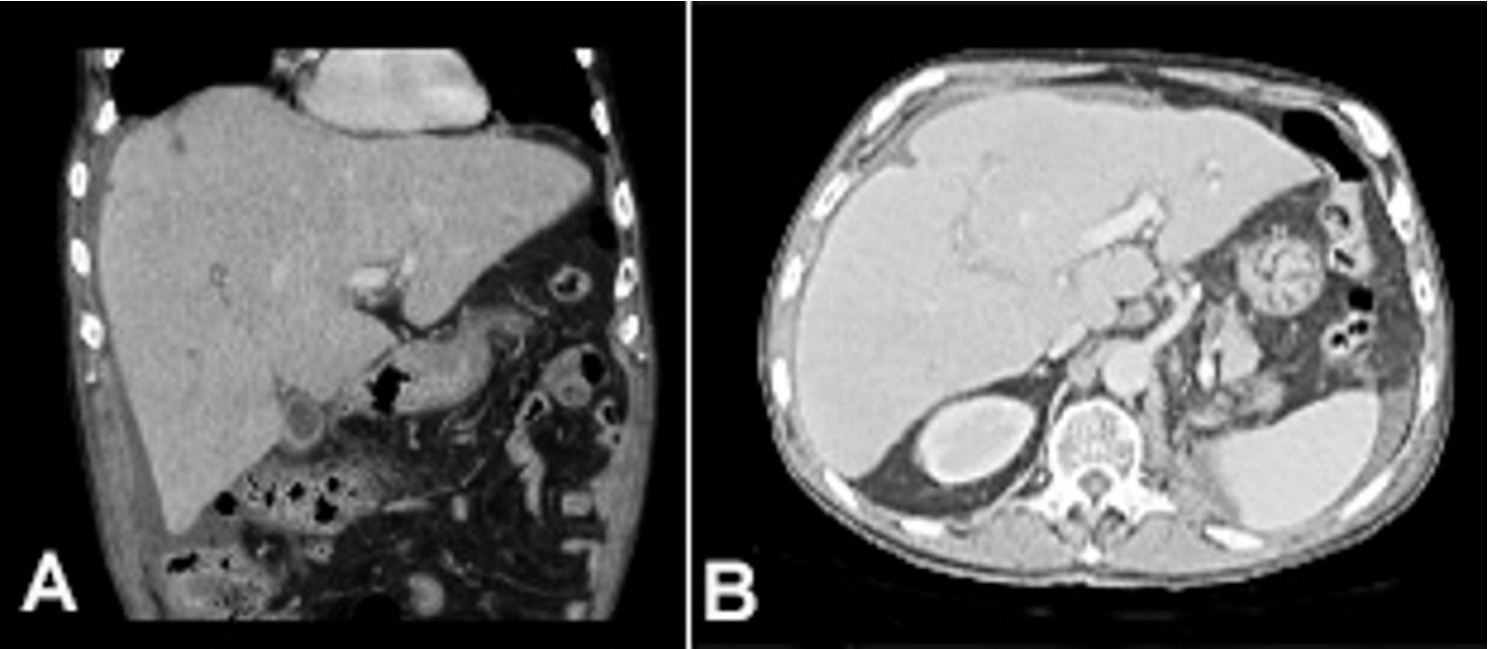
A and B - High-resolution abdominal CT showing hepatomegaly with diffuse inhomogeneous parenchyma, extensive periaortic lymph nodes involvement, and ascites.
